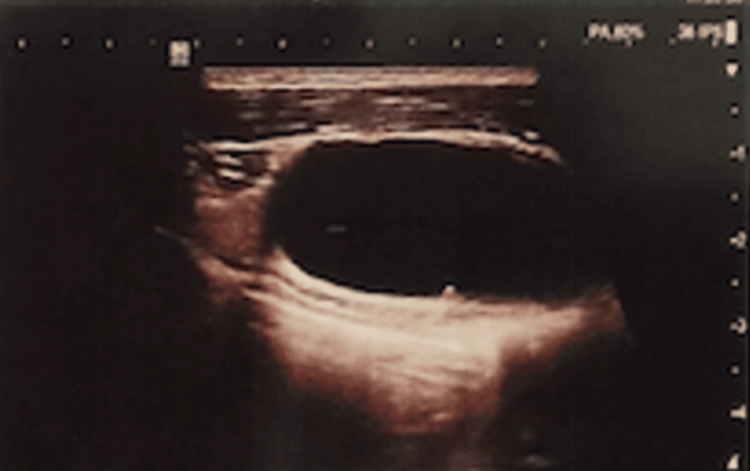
Ultrasound of a cyst with regular walls and transonic content
